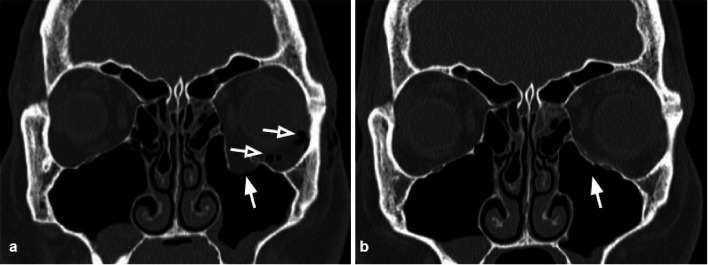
Coronal CT reconstructions. a Blow-out fracture of the left orbital floor (arrow) with herniation of the intraorbital fat into the maxillary sinus. Presence of intraorbital emphysema, an indirect sign of fracture (empty arrows). b Coronal reconstruction of the follow-up CT 2 months after the orbital wall reconstruction through the position of an absorbable mesh. The integrity of the orbital floor is restored (white arrow); the soft tissue herniation is no longer present
